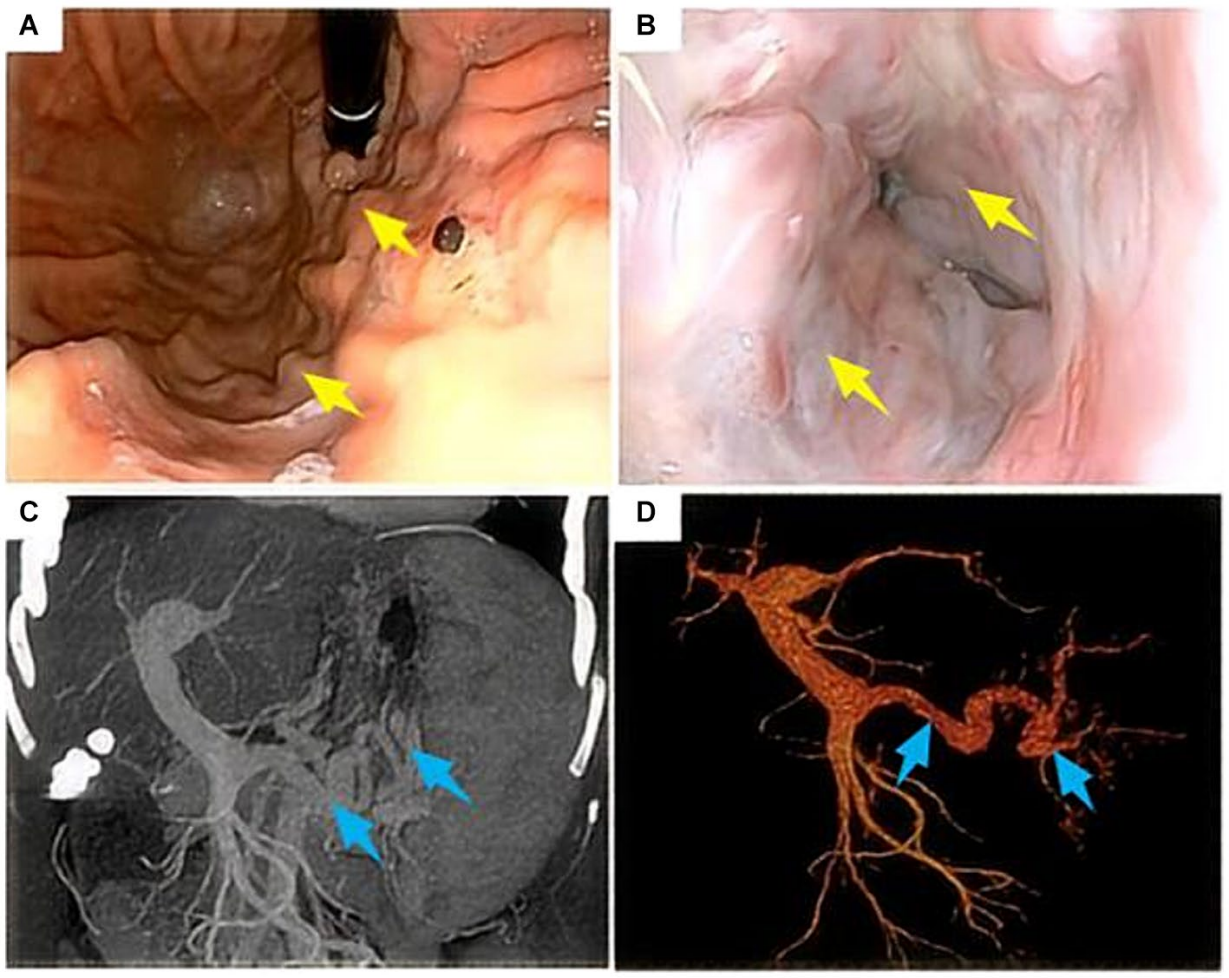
Gastroduodenoscopy and computed tomography scan of the upper abdomen in a 47-year-old female patient who had been vomiting blood repeatedly for 6 years before admission. (A,B) Gastroduodenoscopy suggests severe varices of the esophageal and fundal veins (yellow arrows); (C,D) CT scan of the upper abdomen suggests cirrhosis, splenomegaly, and portal hypertension (widening of the main portal vein and varicose splenic veins) (blue arrows).
